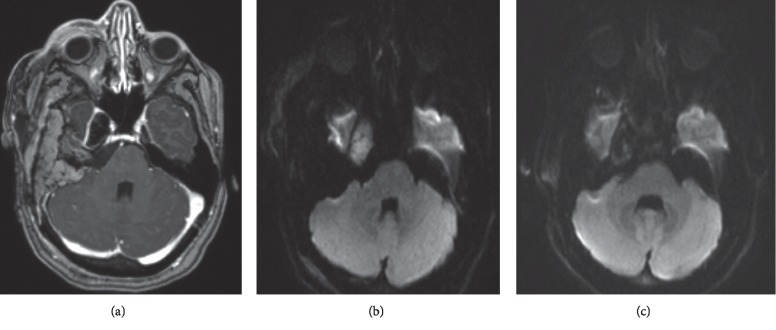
Imaging of patient 2. (a) Preoperative contrast-enhanced axial T1 MRI, showing peripheral enhancement of the cyst wall. (b) Diffusion restriction noted in the lesion preoperatively. (c) Postoperative resolution of diffusion restriction within the lesion.
